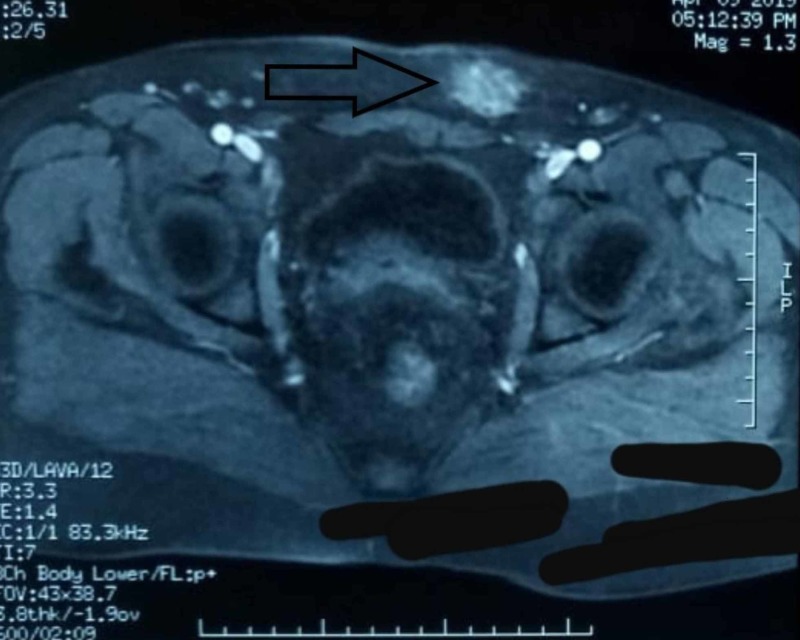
Pelvic magnetic resonance imaging (MRI) scan showing a mass (black arrow) of the left lateral pelvic wall
